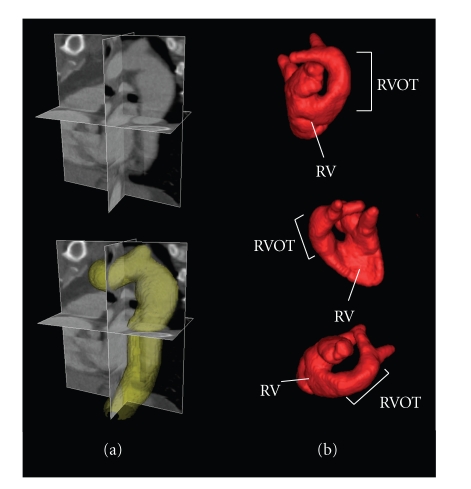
(a) 3D contrast-enhanced CT scan of the aorta (top left), and the same scan with an overlaid 3D segmentation (bottom left) achieved using the Shells and Spheres framework. (b) Surface rendering of a Shells and Spheres segmentation of the right heart with labeled Right Ventricle (RV) and Right Ventricular Outflow Tract (RVOT), shown from three different perspectives.
